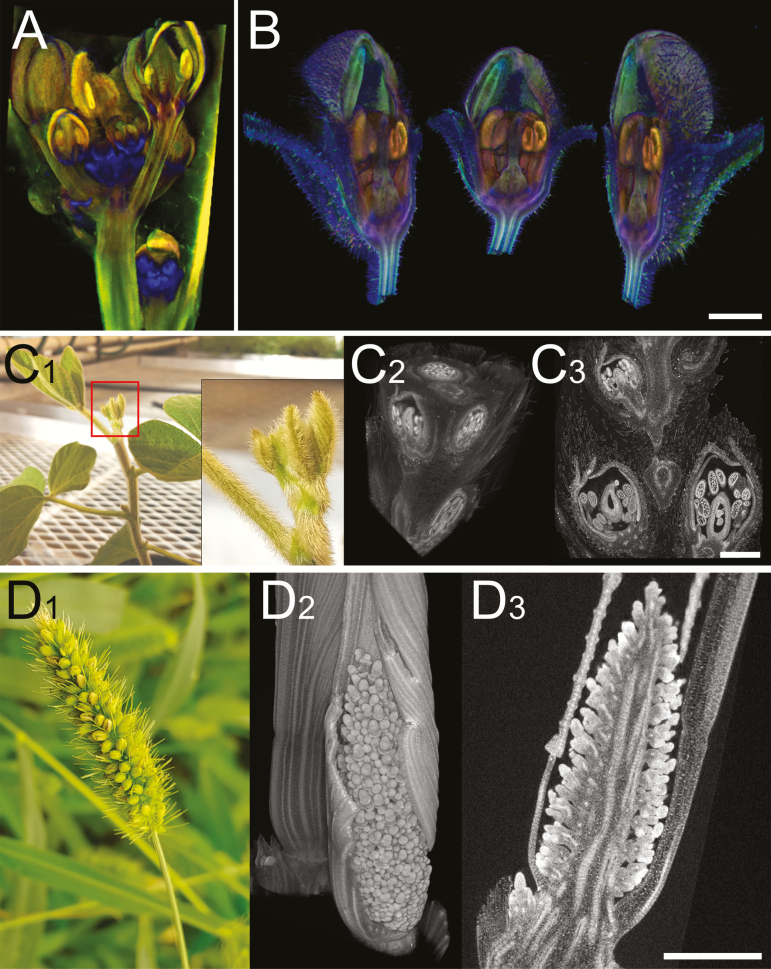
Computed tomography images of flowers. (A) Transmission OPT image of an Arabidopsis inflorescence expressing a GUS reporter for *LEAFY* (blue); image courtesy of Karen Lee. (B) Three views of an Antirrhinum flower imaged with emission OPT and virtual dissecting with a clipping plane to reveal internal structures; image courtesy of Karen Lee. C1–C3. Photograph (C1) and XRM images (C2 and C3) of a young soybean axillary bud containing numerous young florets that will eventually develop into soybean pods; C2 shows a virtual dissection with three clipping planes; C3 shows a computationally reconstructed section. (D1–D3) Photograph (D1), 3D computed reconstruction (D2), and computationally reconstructed section (D3) of young inflorescences from foxtail millet (*Setaria viridis*). Scale bars=500 µm.
